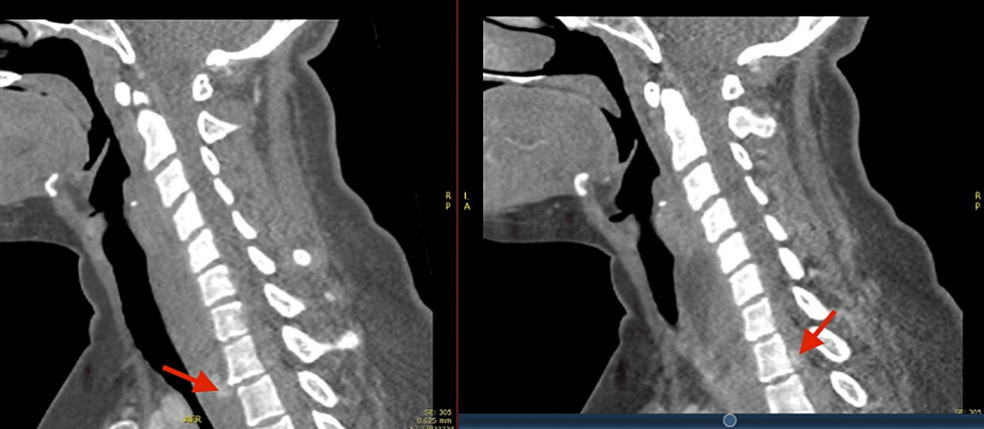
Sagittal soft tissue CT windows reveal a large prevertebral and intraspinal haematoma from level C5-T2, with specks of contrast extravasation (Red arrow).
